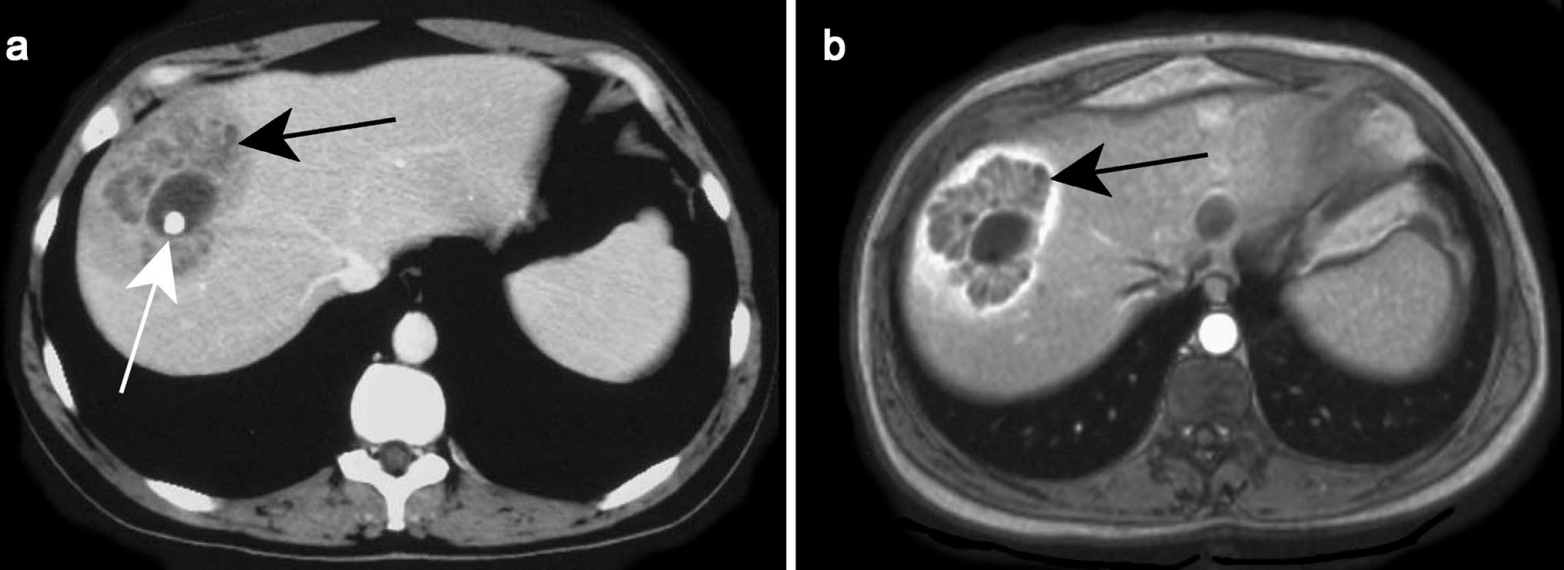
Brucellosis in a 42-year-old male patient with evening fever and sweating. Contrast-enhanced axial CT image a shows a heterogeneous lesion with enhanced contours (black arrow), showing a central hypoechoic fluid component and a calcium deposit (white arrow). Axial contrast-enhanced T1-weighted image b shows enhancement of the peripheral tissular areola (black arrow) and central saccular formation with fluid, surrounded by an intermediary heterogeneous component. Reprinted from Sisteron et al. [27], with permission from Elsevier
